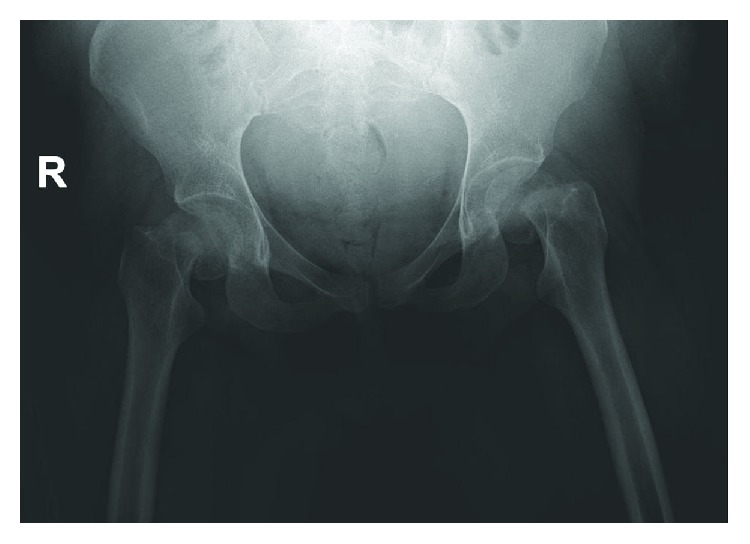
Preoperative anterior-posterior radiographs of the pelvis demonstrating the displaced bilateral femoral neck fractures.
